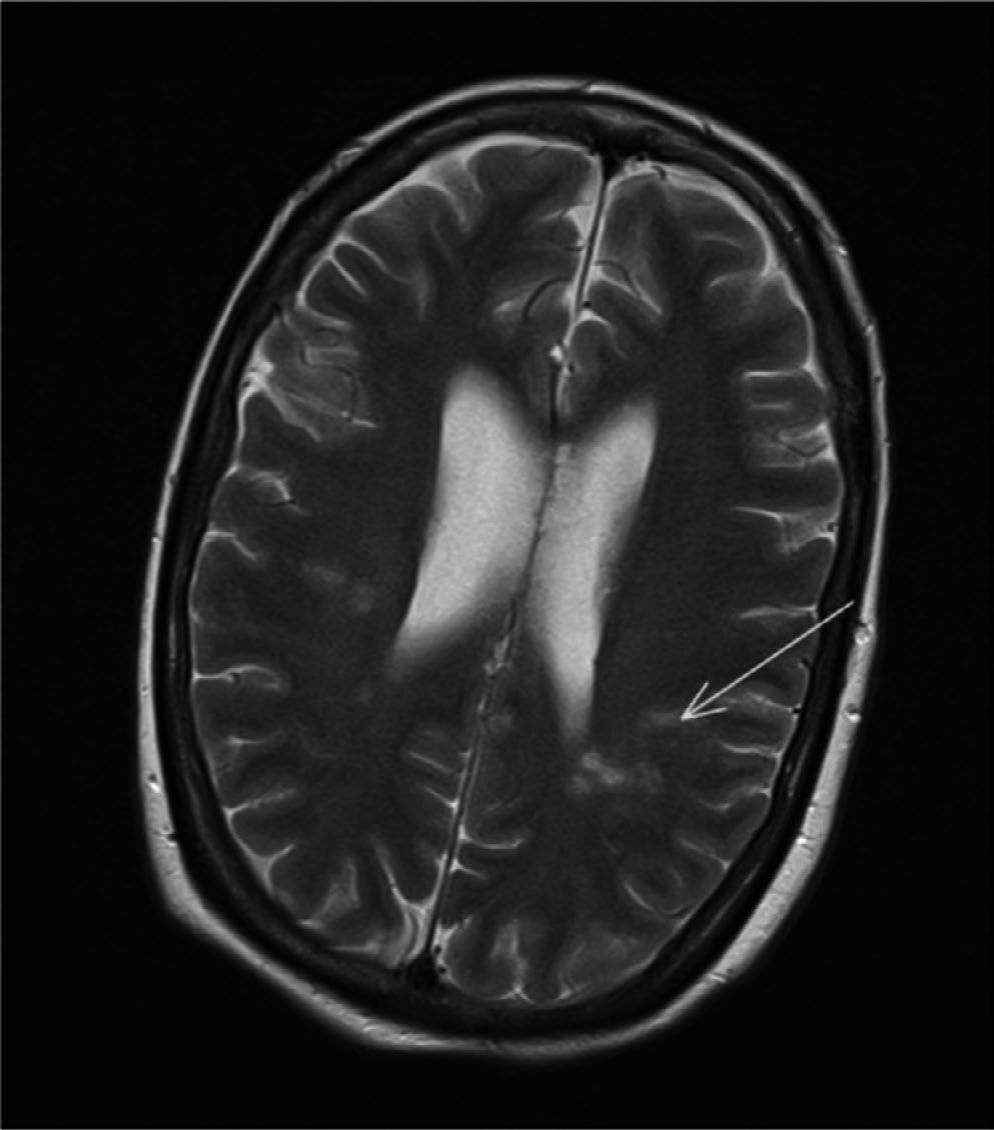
MRI head with contrast: axial T2 image. Arrow shows area of increased signal in middle cerebral artery territory indicating acute infarction
